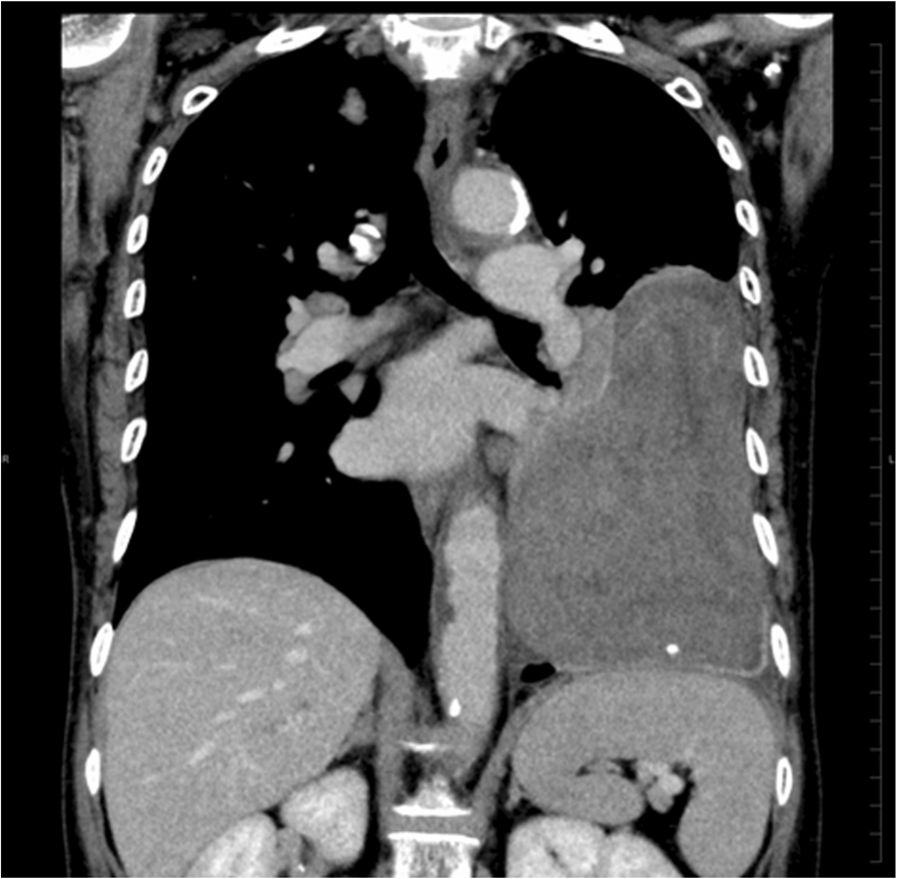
Chest computed tomography reveals a mass measuring 15 cm with no invasion to the thoracic wall
